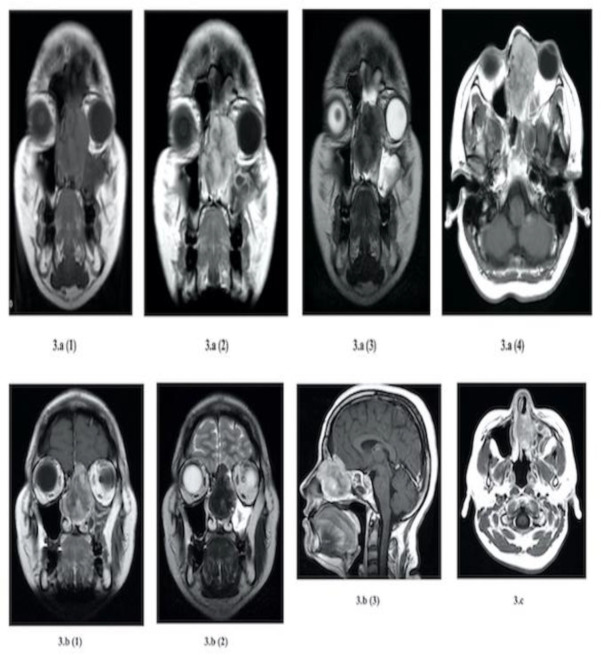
MRI Brain and PNS images of mass extensions into Orbit and Intracranially (extradural)
